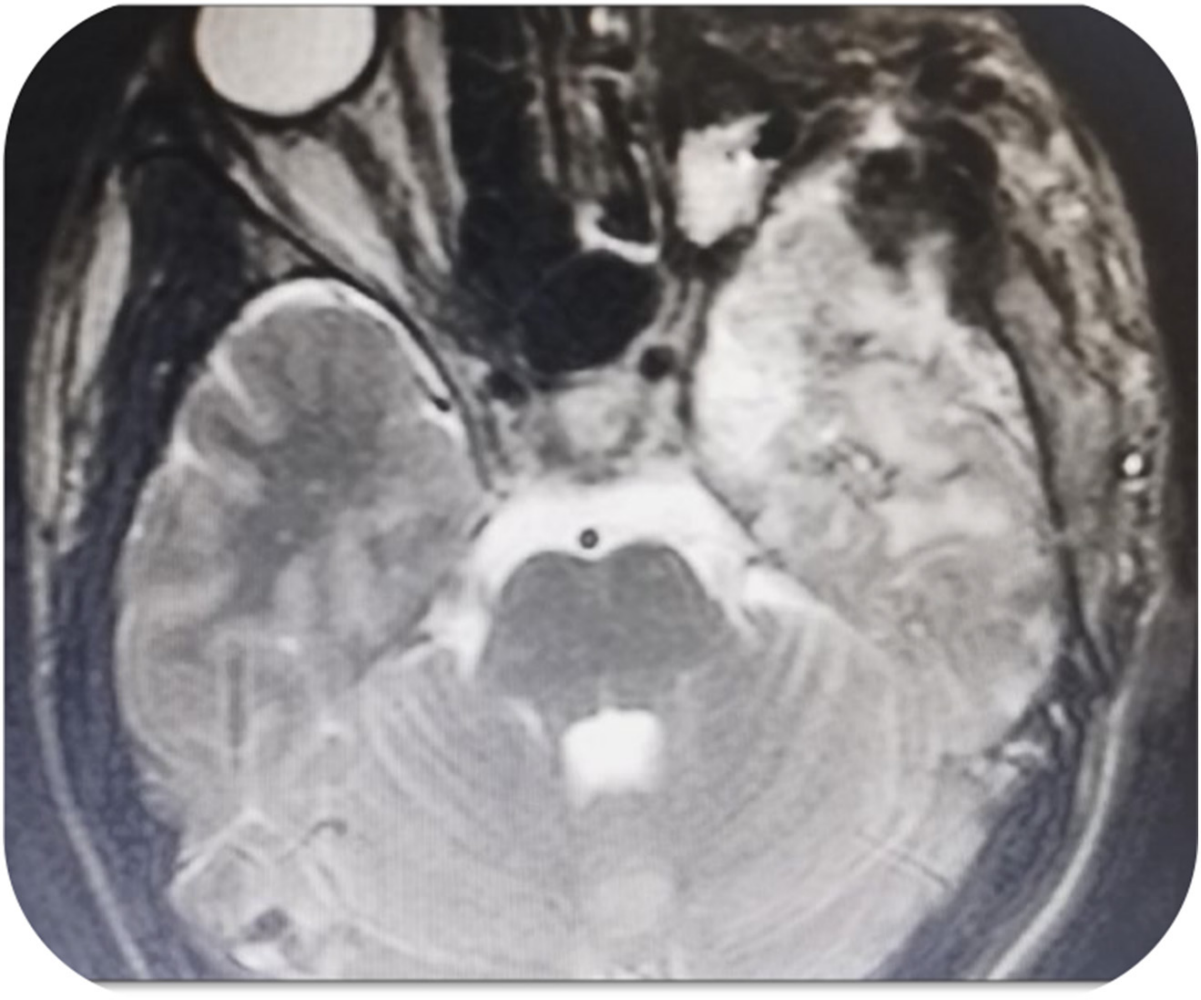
Brain MRI after frontotemporal‐orbit zygomatic (FTOZ) surgery and after resection the mass.
